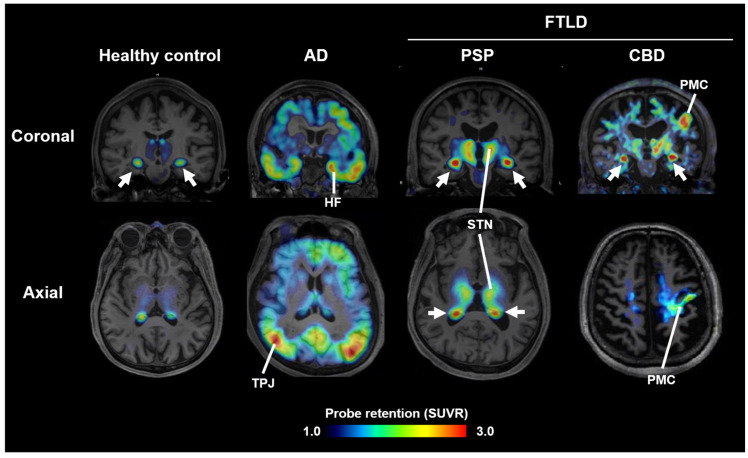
Tau PET images acquired with ^18^F-florzolotau in healthy controls and cases with AD, PSP, and CBD. Arrows indicate non-specific radioactivity accumulations in the choroid plexus. HF, hippocampal formation; STN, subthalamic nucleus and neighboring thalamic structures; PMC, primary motor cortex; TPJ, temporoparietal junction; SUVR, standardized uptake ratio at 90–110 min after tracer injection. Modified from Ref. [9].
